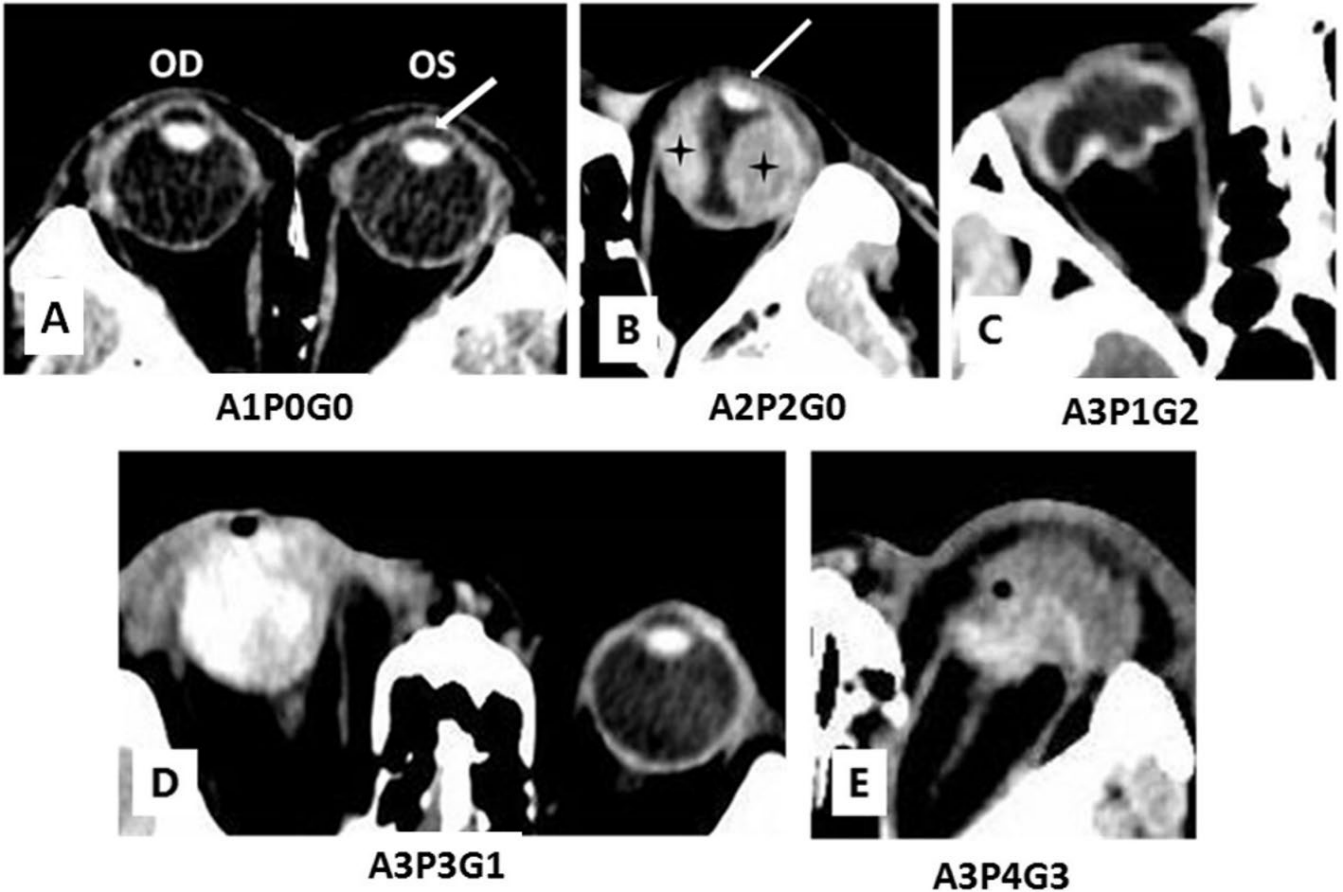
A Left open-globe injury after being hit in the left eye with a sheet metal. It was a 6 mm area 1 wound. Axial CT scan of the eyes shows relative shallow anterior chamber depth (arrow) of the left globe compared to that of the right eye. B Left open-globe injury after being hit in the left eye with metal block. It found to be a 20 mm wound involving area 1 and 2 during suture surgery. Axial CT scan of the eyes shows relative shallow anterior chamber depth of the left globe compared to that of the right eye. The patient was lost follow-up. C Right open-globe injury after hitting on the door. It was a 12 mm area 1 wound. Axial CT scan of the eyes shows the absence of the lens. It was found that both lens and iris were absent during suture surgery. Volume of the globe reduced to about half of normal. Fortunately, there was not massive hemarrhage in the vitreous chamber. After suture surgery, the final visual acuity achieved 0.1 and no further surgery was carried out during over 3 years of follow-up. D Right open-globe injury after being hit in the right eye by an exploding glass bottle. Axial CT scan of the eyes shows the globe is full of massive hemarrhage. It found to be a 25 mm wound involving area 1, 2 and 3 during suture surgery. After suture surgery, the injured eye became phthisical eventually during follow-up. E Left open-globe injury after being hit by exploding fireworks. It found to be a 35 mm wound involving area 1, 2 and 3 during suture surgery. Forty five days later, the injured eye was eviscerated
